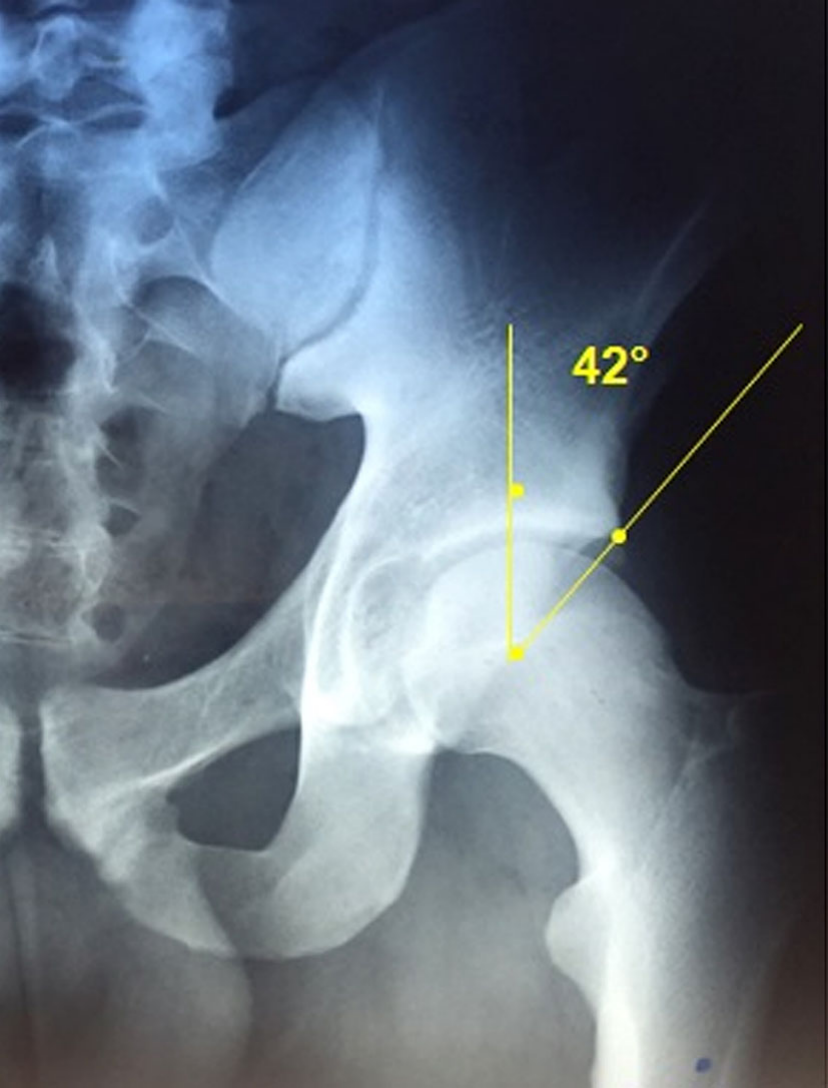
Centre-edge angle (CEA) measurement on an AP pelvis X-ray. The CEA is the angle between the line connecting the centre of the femoral head and the acetabulum and the line perpendicular to the line connecting the sit bones of the ischial tuberosity. A value of ≥40° is consistent with a pincer morphology
